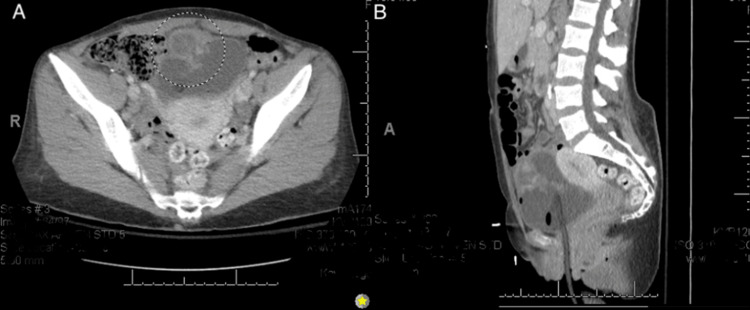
Contrast-enhanced CT of the pelvis (axial and sagittal views). (A) Axial CT image of the pelvis showing an anterior dome bladder mass (dotted circle) measuring approximately 6.2 cm. (B) Sagittal CT image of the pelvis confirming the intravesical location and extent of the bladder mass.
